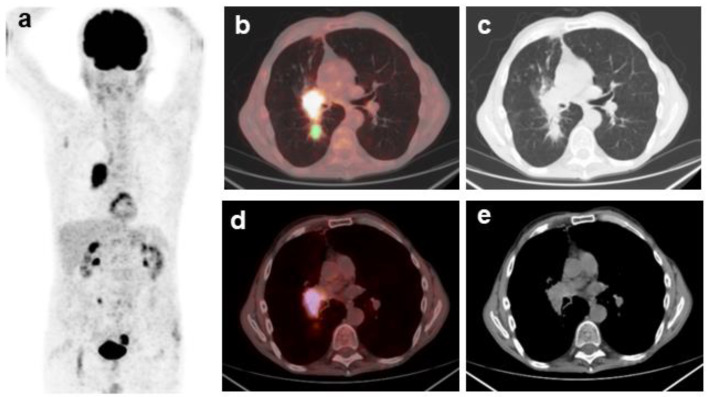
Representative images of ^18^F-FDG PET/CT scan in a patient with stage IIIA non-small cell lung cancer. (a). Maximal intensity projection PET image; (b). transaxial fusion image of co-registered PET and CT showing high FDG uptake in the primary lung tumor and hilar lymph node. A tridimensional region of interest was drawn around the primary lung tumor (green); (c). corresponding CT image of the thorax using window for lung parenchyma visualization; (d). transaxial fusion image of co-registered PET and CT showing the FDG avid metastatic hilar lymph node. A tridimensional region of interest was drawn around the targeted lymph node (pink); (e). corresponding CT image of the thorax using window for mediastinal visualization.
